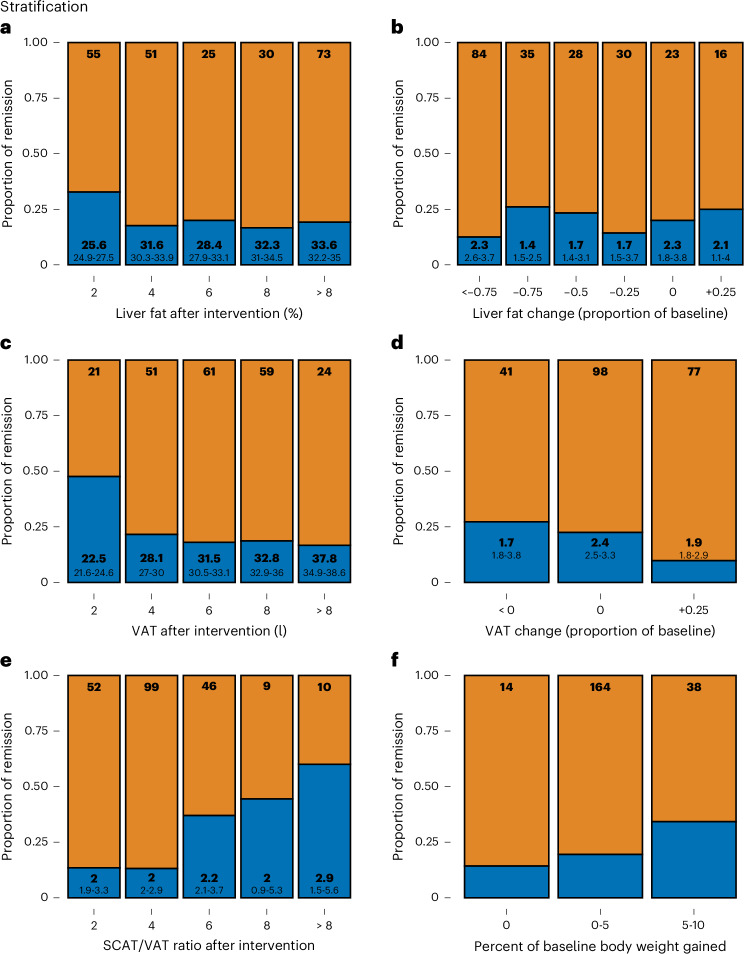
Stratification of prediabetes remission. a, Stratification of R (defined by NGR, that is, prediabetes remission, after 12 months of LI, *n* = 51; blue) and NR (that is, not reaching NGR, *n* = 183) by postintervention hepatic lipid content as assessed with ^1^H-MRS. b, Change of hepatic lipid content during LI. c, Postintervention VAT volume as assessed with MRI. d, Change of VAT volume during LI. e, SCAT/VAT ratio. f, Change of body weight during LI. Top numbers inside the bars indicate stratum size (*n*). Large bottom numbers indicate BMI (a,c) or percent body weight change (b,d,e). Small bottom numbers indicate 95% CI, respectively.
